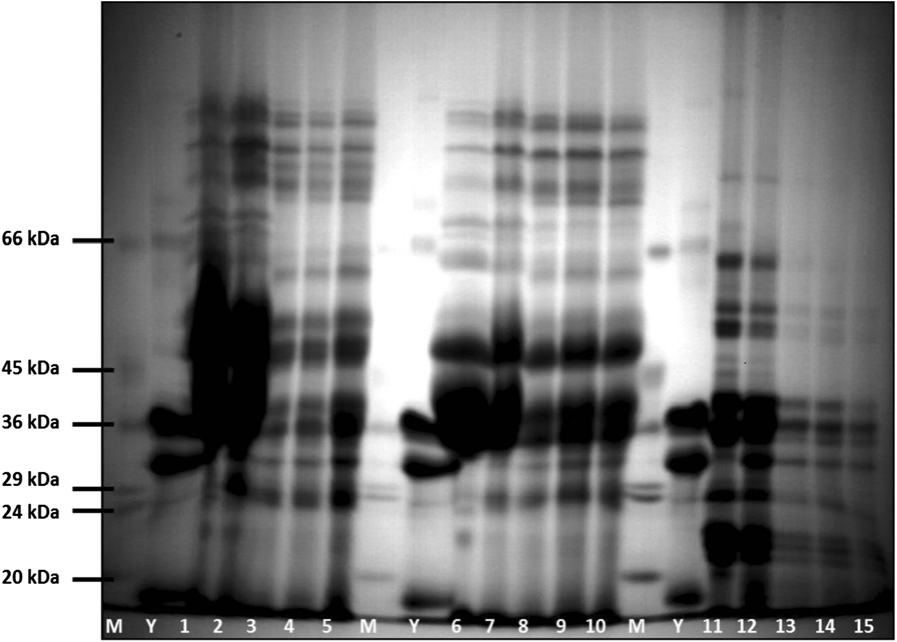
SDS-PAGE patterns of the gliadin and prolamin fractions. M: Marker, Y: Yoghurt, 1: WF, 2: WFT day 0, 3: WFT day 1, 4: WFT day 2, 5: WFT day 3, 6: WWF, 7: WWFT day 0, 8: WWFT day 1, 9: WWFT day 2, 10: WWFT day 3, 11: CF, 12:CT day 0, 13: CT day 1, 14: CT day 2, 15: CT day 3.
